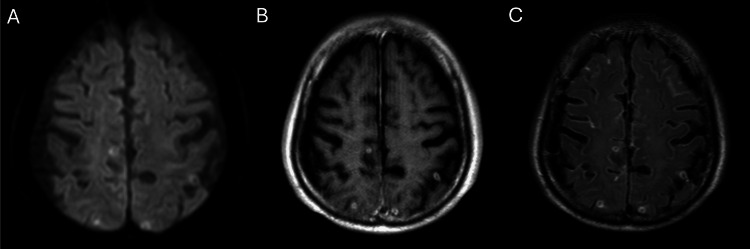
Cerebral magnetic resonance imaging. Cerebral magnetic resonance imaging demonstrating multiple nodules with ring-like enhancement (target sign). (A) Diffusion-weighted imaging. (B) Gadolinium-enhanced T1-weighted imaging. (C) Contrast-enhanced fat-saturated fluid-attenuated inversion recovery imaging.
